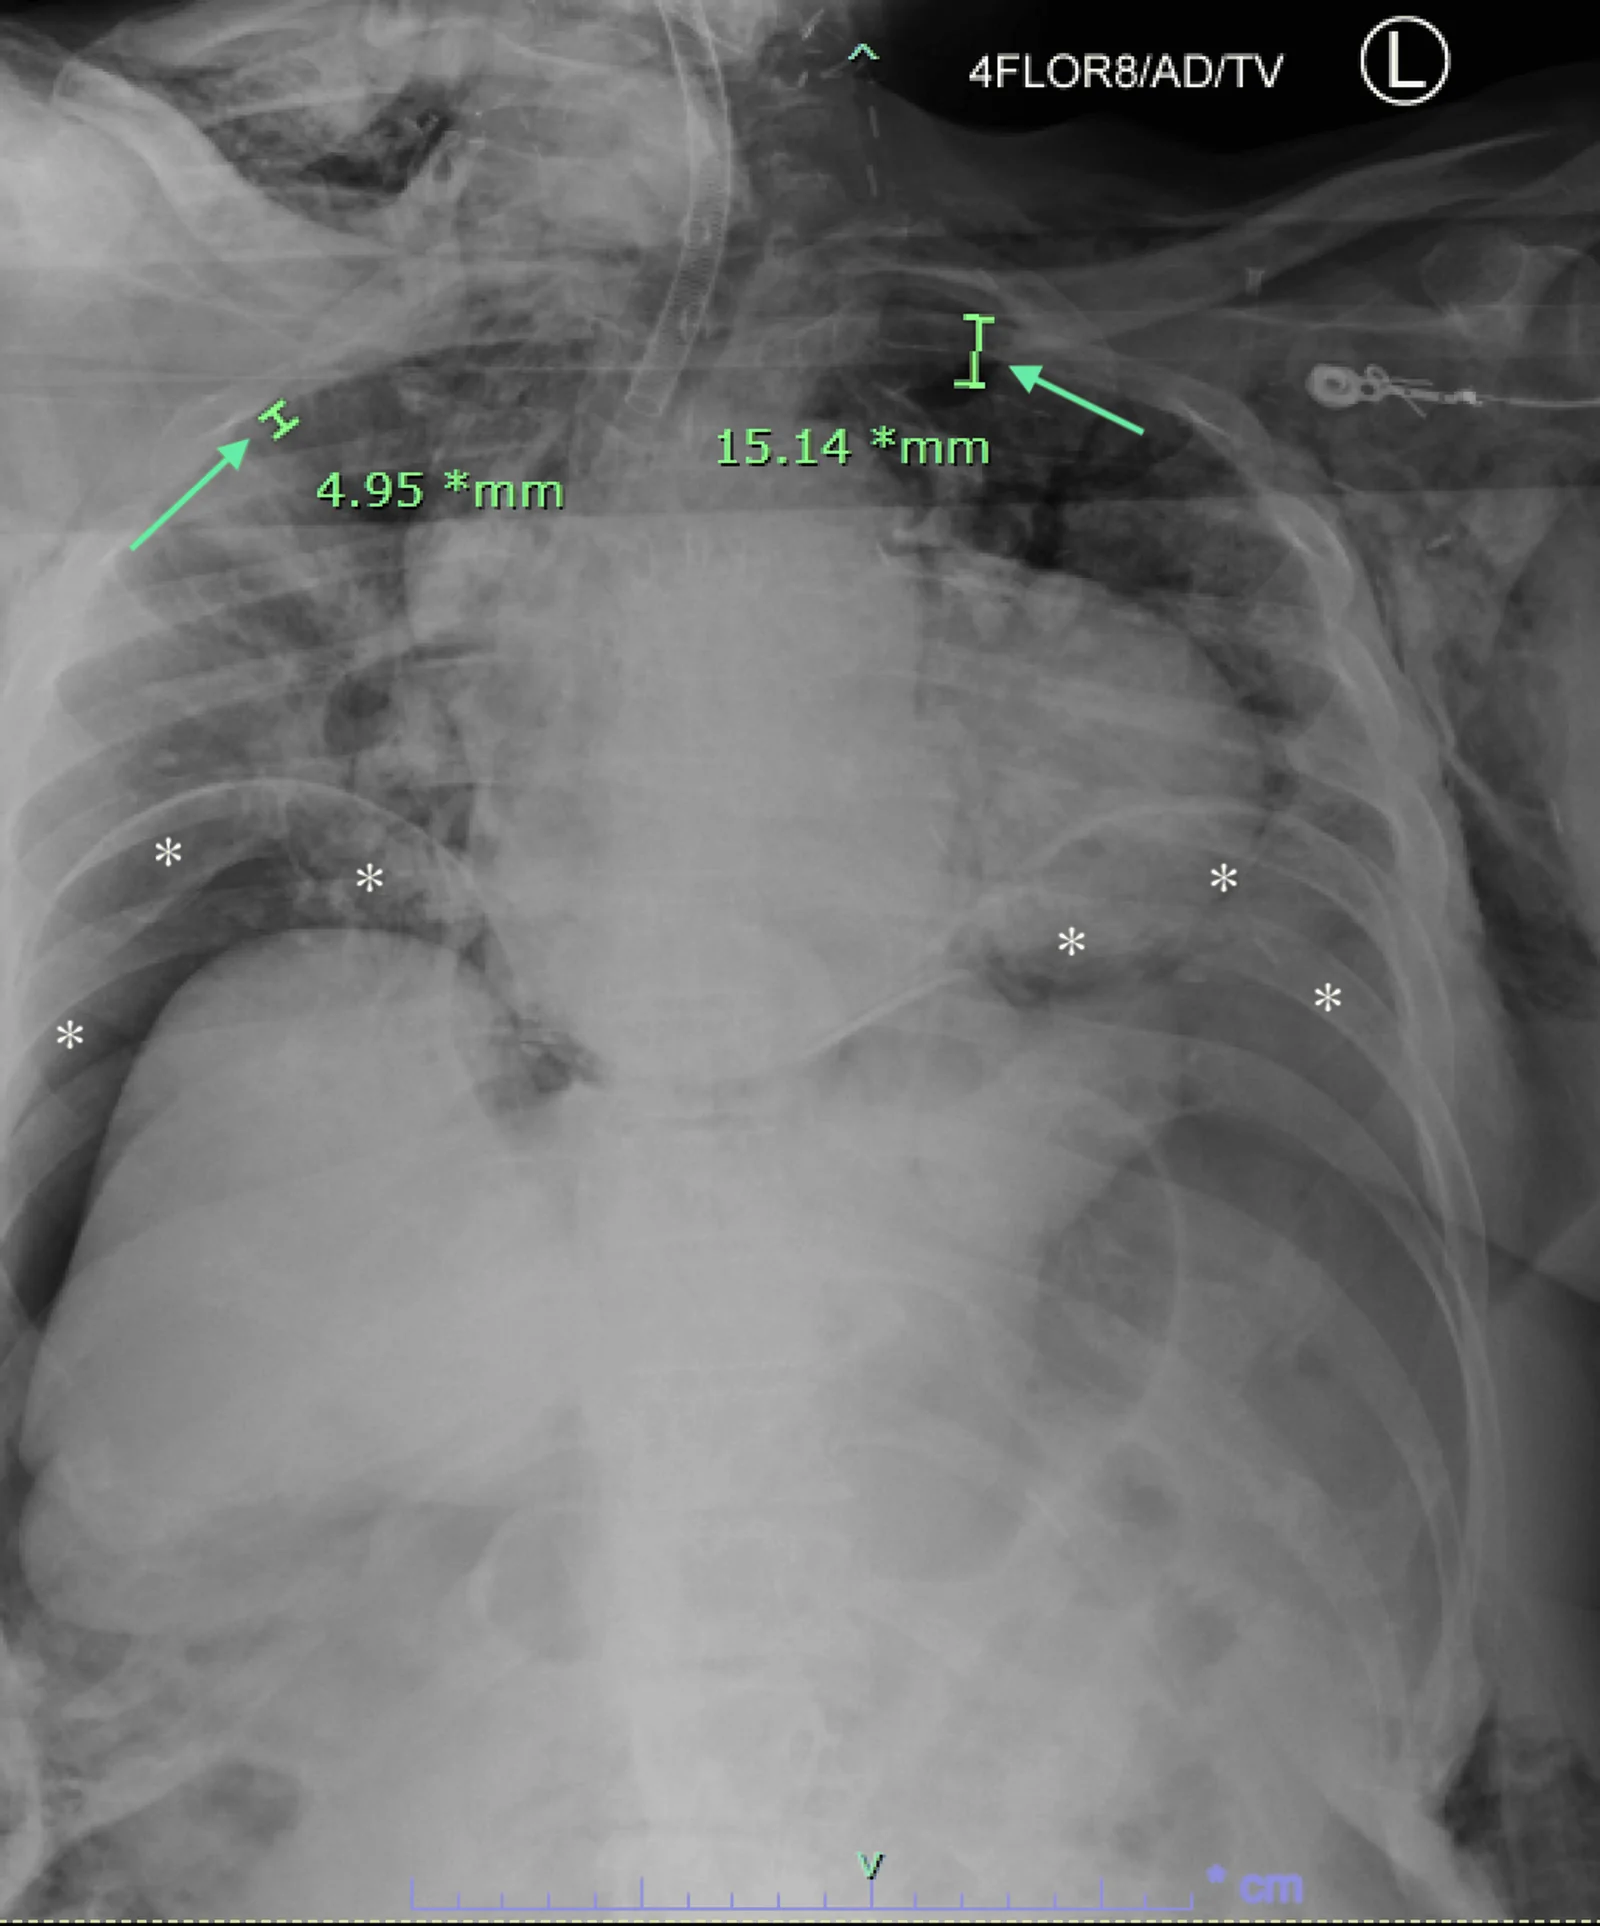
Chest radiograph Showing small bilateral pneumothoraces (green arrows) and extensive pneumoperitoneum (white stars).
